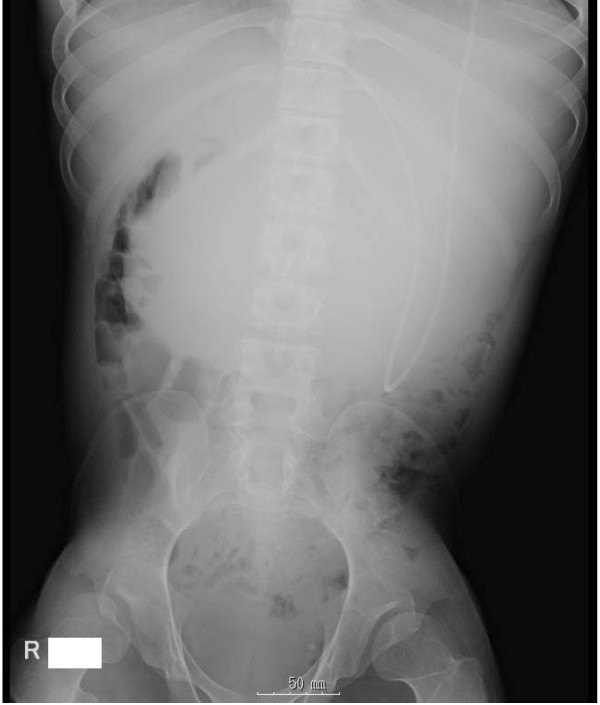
Abdominal radiography showing the VPS catheter and a soft-tissue mass located in the upper abdomen.
